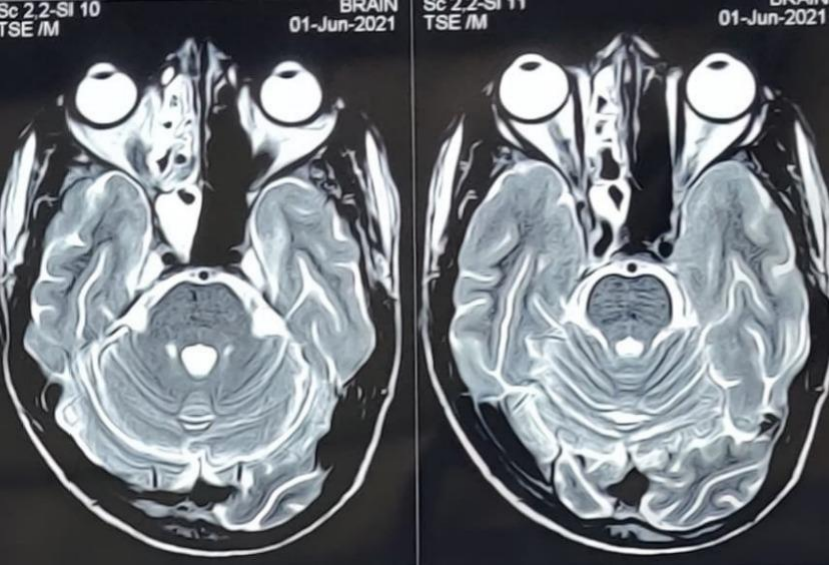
Axial section of MRI showing thickened optic nerve sheath in the right eye and involvement of the right ethmoidal air cells
